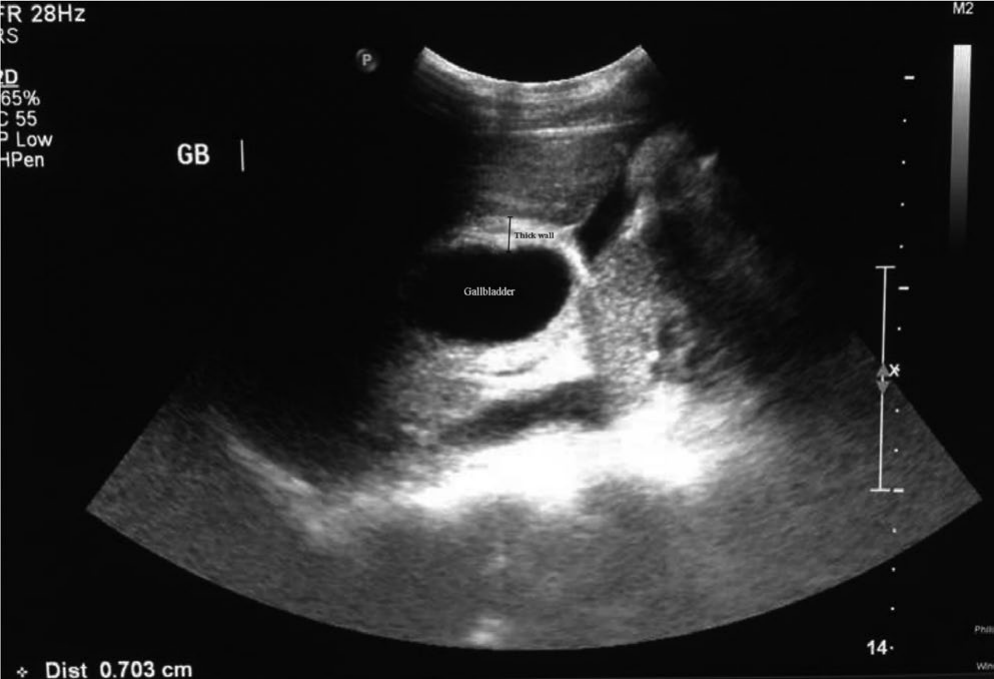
Ultrasound appearance of gallbladder volvulus: note significant gallbladder wall thickness without stones
